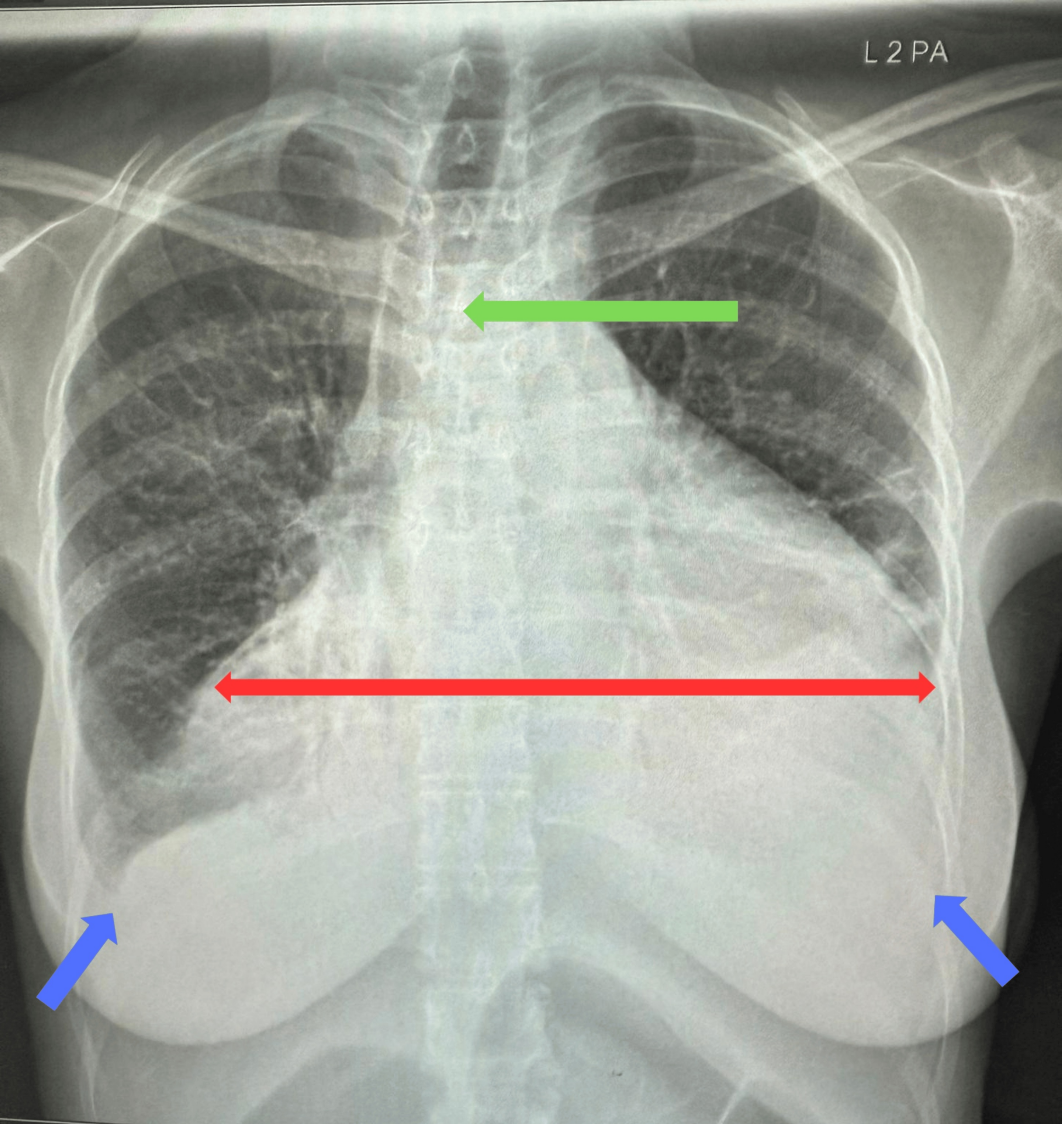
Chest X-ray showing cardiomegaly (Red), bilateral blunt costophrenic angles (Blue), and deviated trachea (Green).
